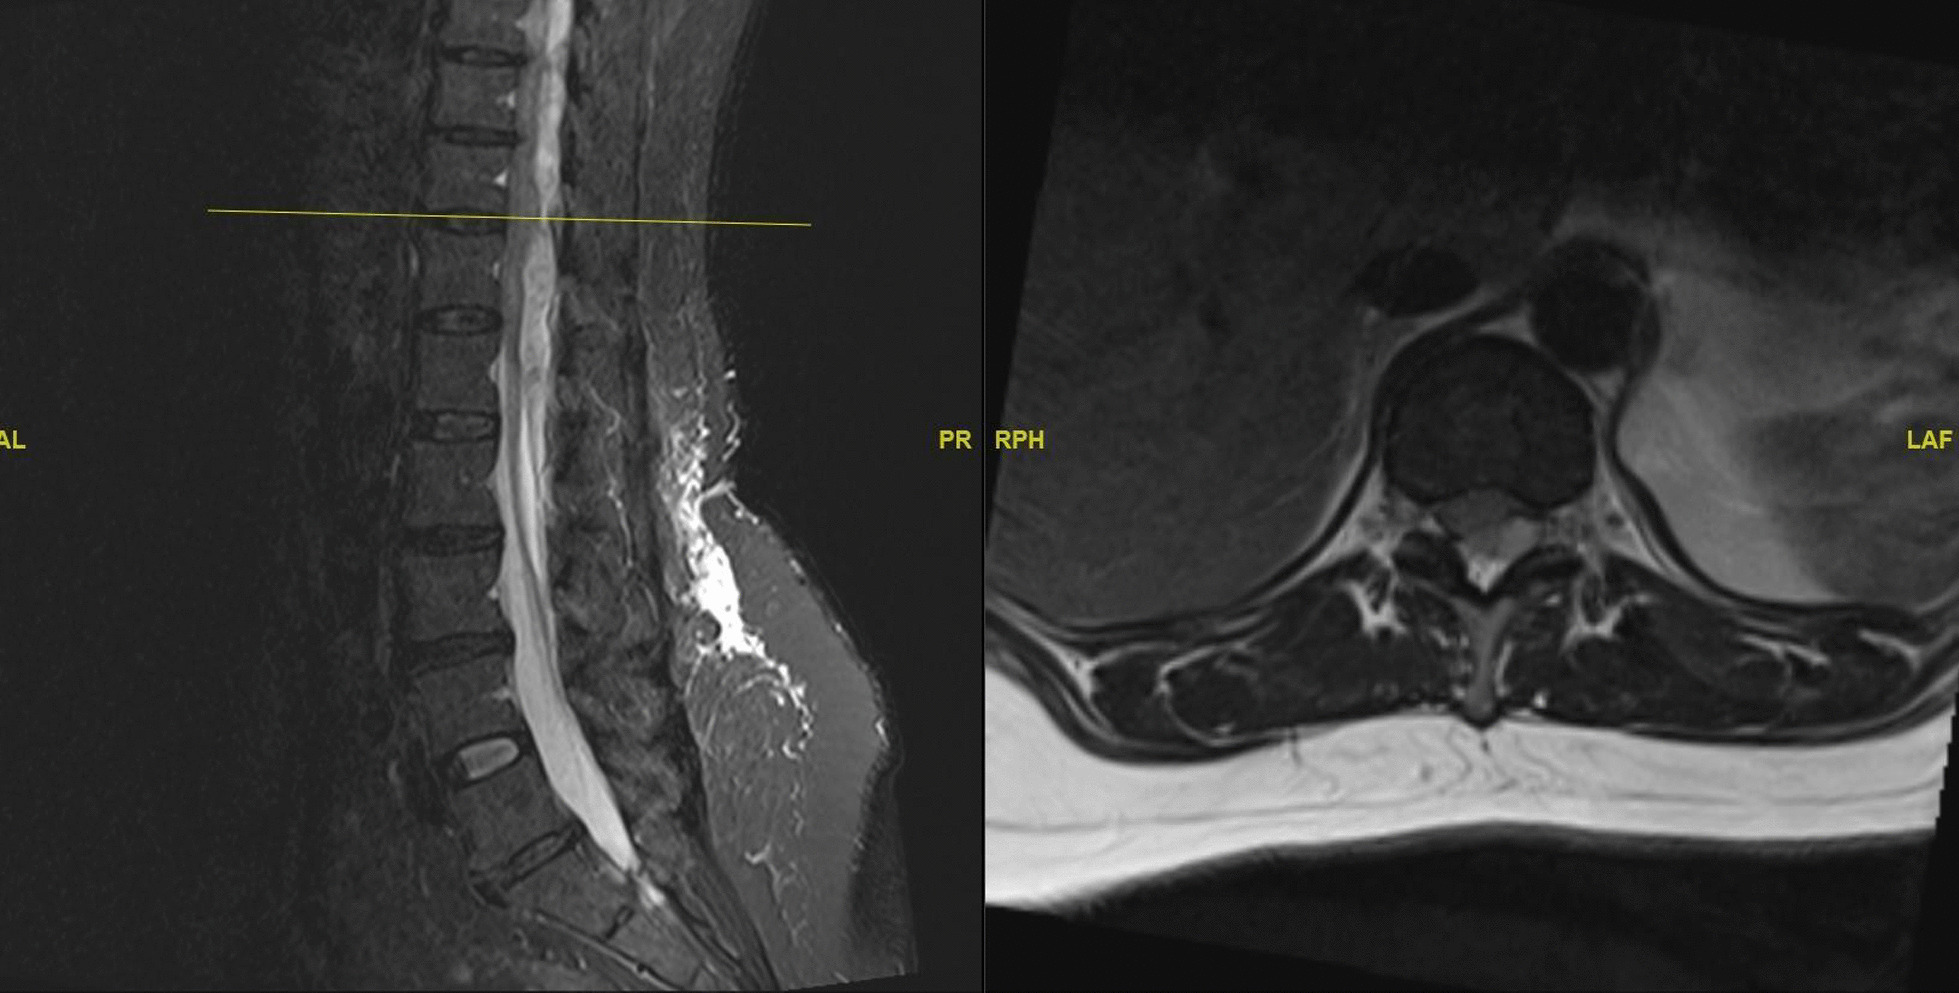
Pre-operative MRI whole spine
